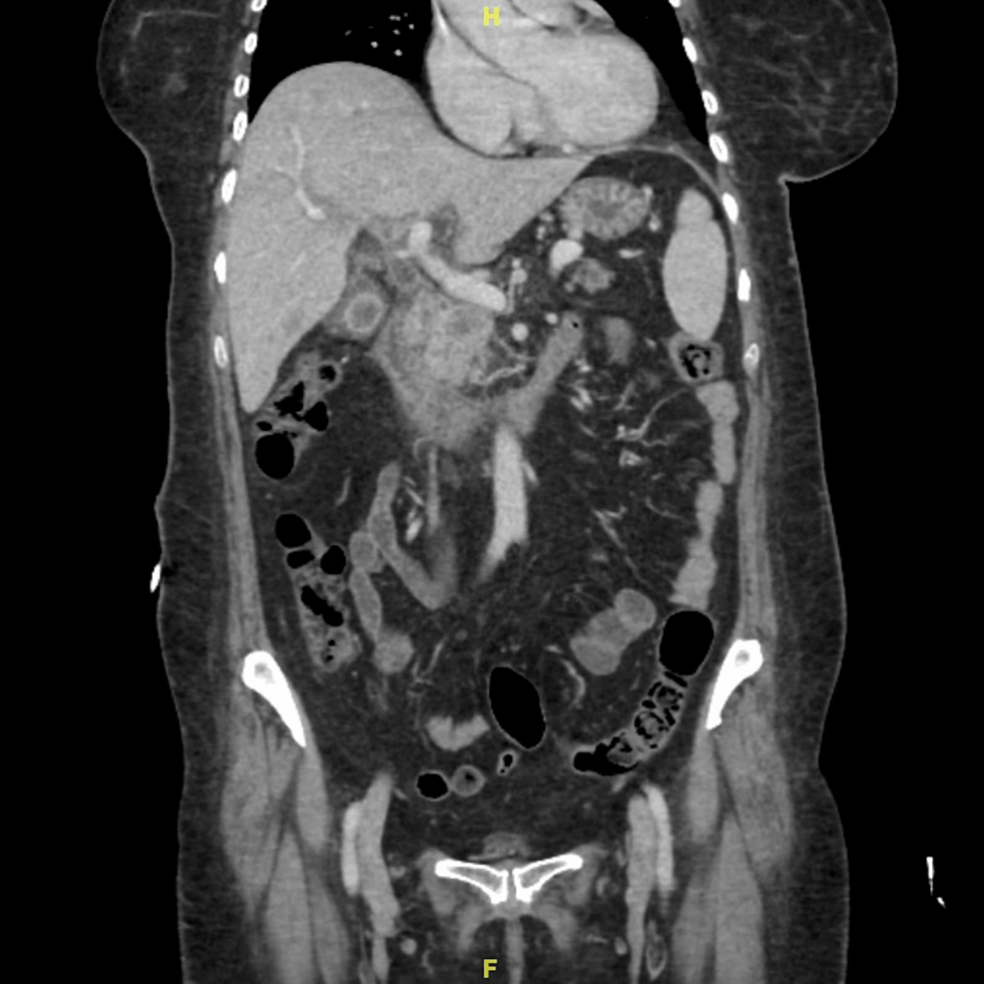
Coronal CT for patient 2 Coronal CT of abdomen and pelvis shows a pancreatic head mass.
